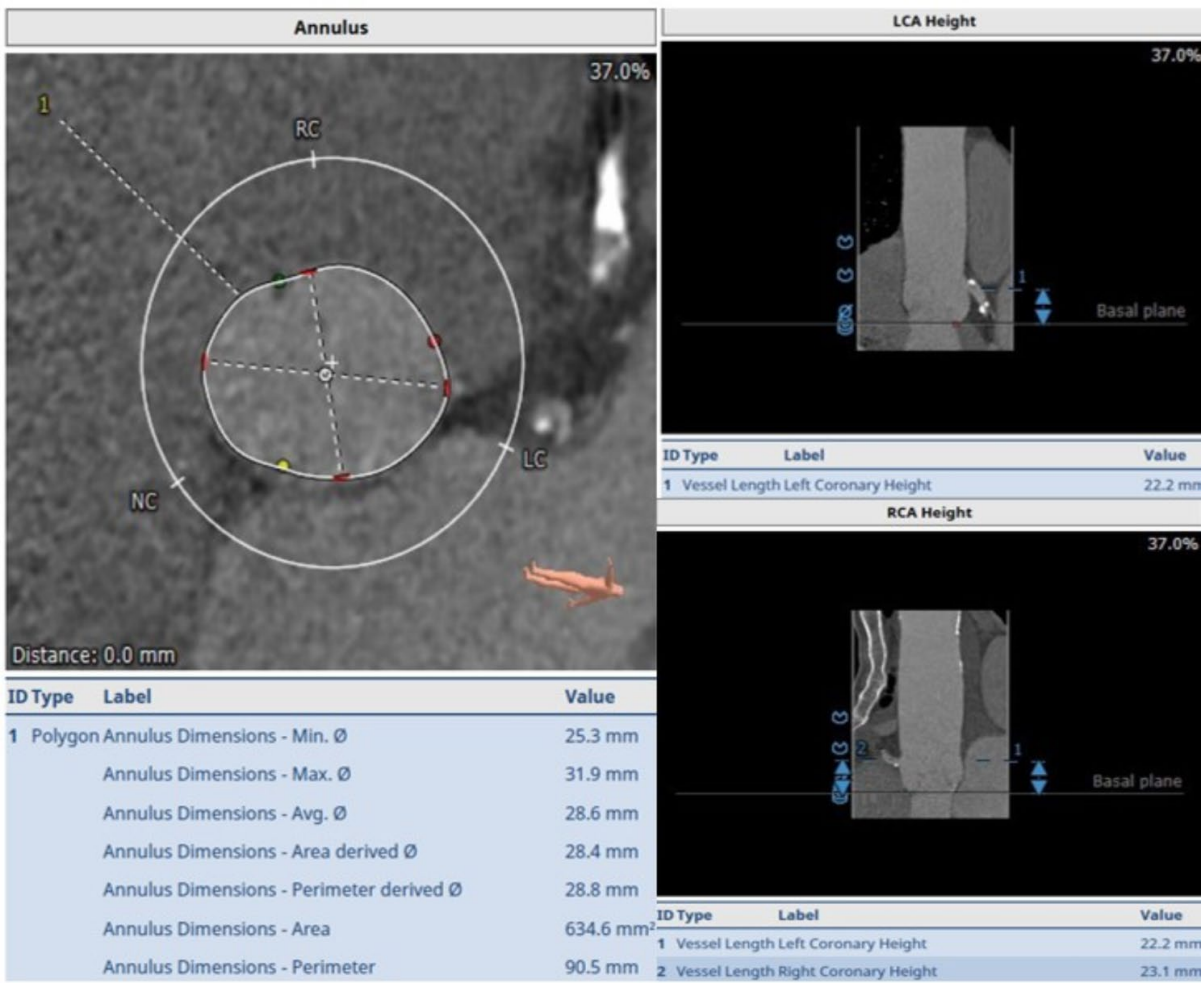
Annulus measurements on CT scan preoperatively
